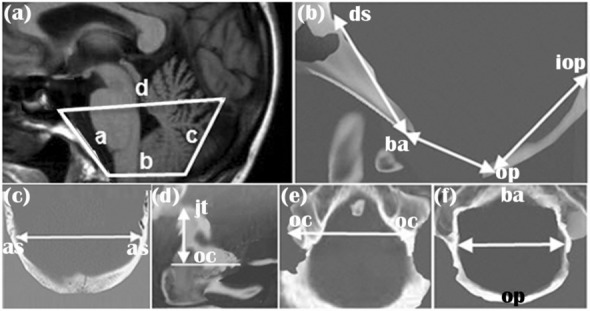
Panels show the measurements of PCF made on T1-weighted MRI. (a) a: clivus; b: foramen magnum; c: supraocciput; d: Twining line. (b) Distance from the opisthion (op) to the inner surfaces of the basion (ba) is the anterior–posterior diameter of the foramen magnum; distance from the opisthion to the center of the internal occipital protuberance (iop) is the axial length of supraocciput; and distance from the basion to the top of the dorsum sellae (ds) is the axial length of clivus. (c) Shown the distance between asterions (as). (d) The perpendicular distance from the top of the jugular tubercle (jt) and the bottom of the occipital condyle (oc) along a line parallel with the orbitomeatal line is the axial height of condyle. (e) Distance between outer surfaces of occipital condyles (oc) is the width of condyles. (f) Distance between opisthion (op) and the basion (ba) is the maximum transverse diameter of the foramen magnum.
